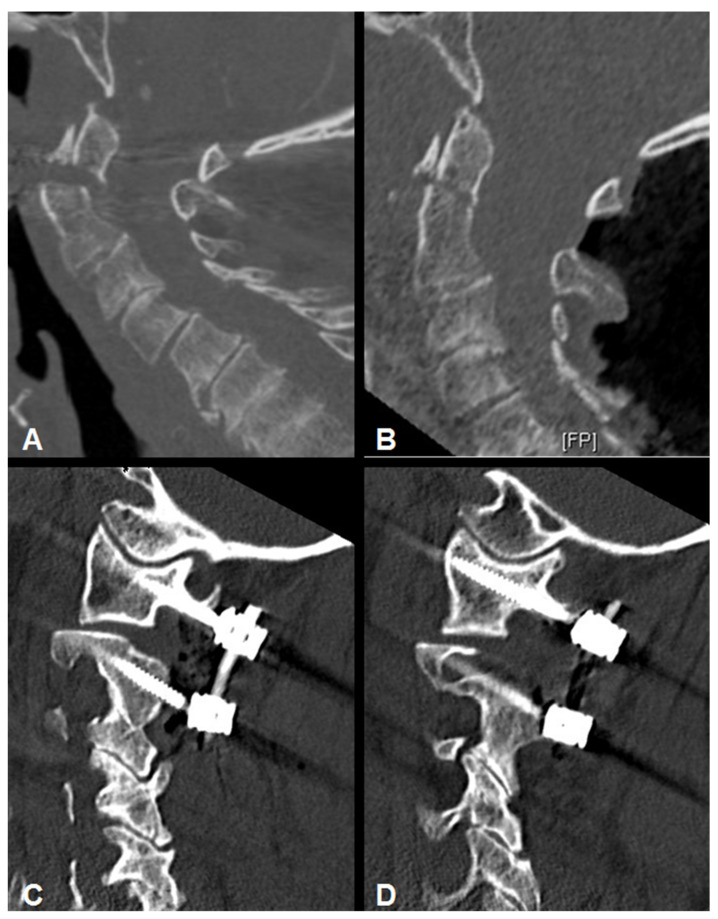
(A) Sagittal CT-image of a 91-year old male with an unstable odontoid type II fracture. (B) Intraoperative Sagittal CT data set for spinal navigation showed complete fracture reduction. Postoperative sagittal CT reconstruction after atlanto-axial stabilization using the posterior arch C1 lateral mass (PALM) technique (C,D).
